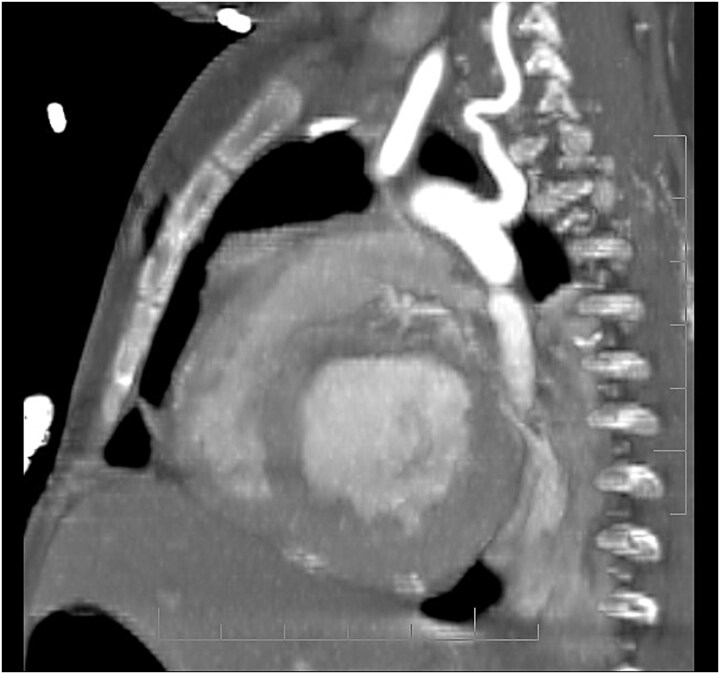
Three-dimensional CT reconstruction showing the aortic arch with coarctation (narrowing) and the ARSA originating from the descending aorta distal to the coarctation site.
